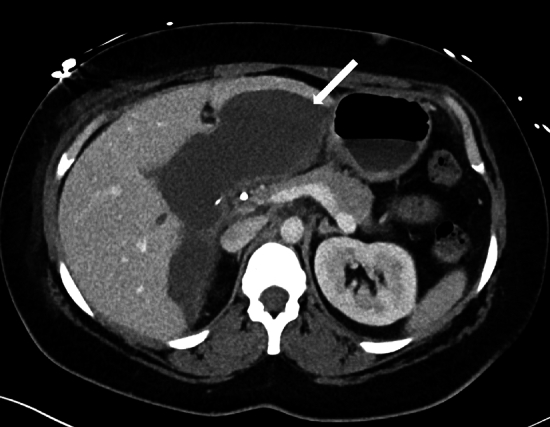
A 29-year-old female presenting with postoperative pain, tachycardia, and leukocytosis post cholecystectomy with subcapsular hepatic hematoma. An axial CECT image of the abdomen performed 2 weeks after surgery demonstrates a large subcapsular hepatic collection indenting the left hepatic lobe (arrow). Drainage of the collection showed serosanguinous fluid consistent with hematoma. An MRI of the abdomen using Eovist contrast was also performed, which did not show a bile leak (not shown)
